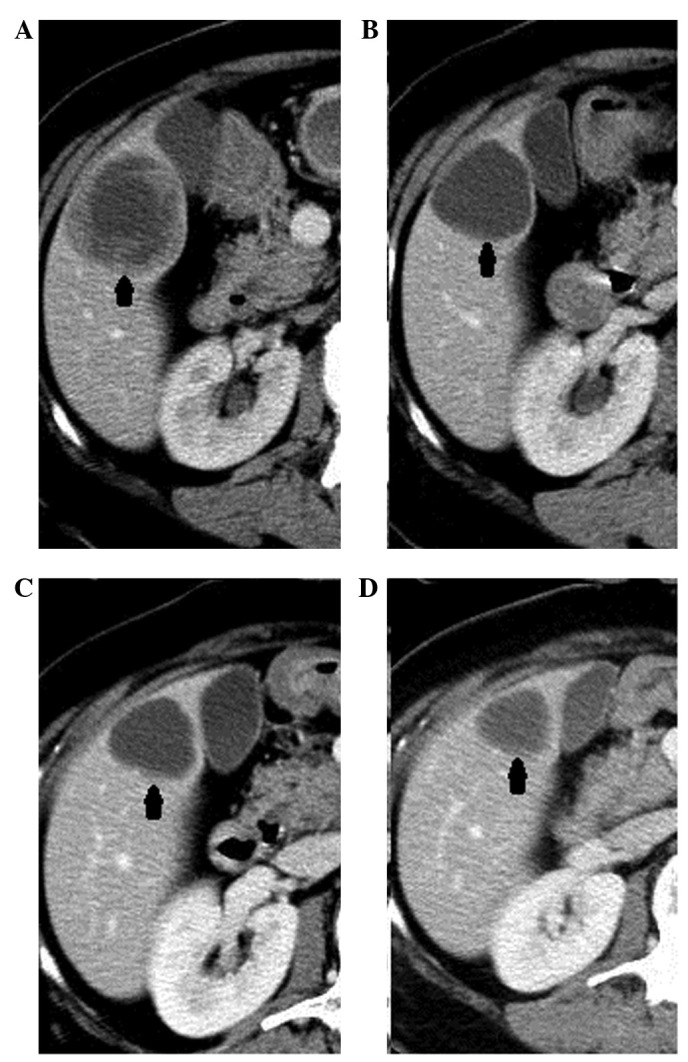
Enhanced CT scan of liver metastasis (arrow) before imatinib treatment (A), and (B) 2, (C) 4 and (D) 6 months after therapy revealed a significant decrease in the tumor size and CT value. CT, computed tomography.
